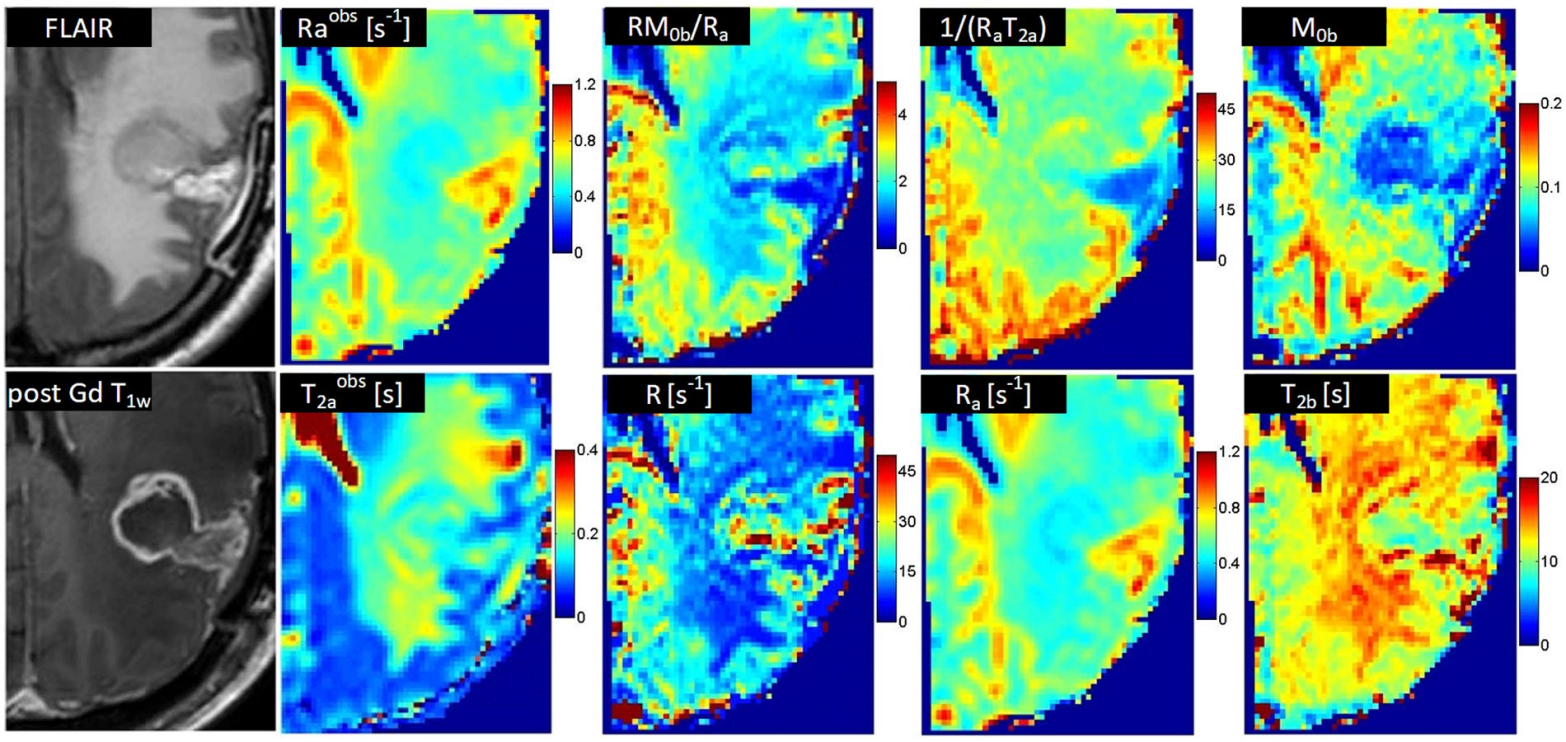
The FLAIR and post-Gd T_1w_ images of the tumor for a representative patient, as well as parametric maps of R_a_^obs^, T_2a_^obs^, and qMT parametric maps corresponding to RM_0b_/R_a_, R, 1/(R_a_T_2a_), M_0b_, and T_2b_.
